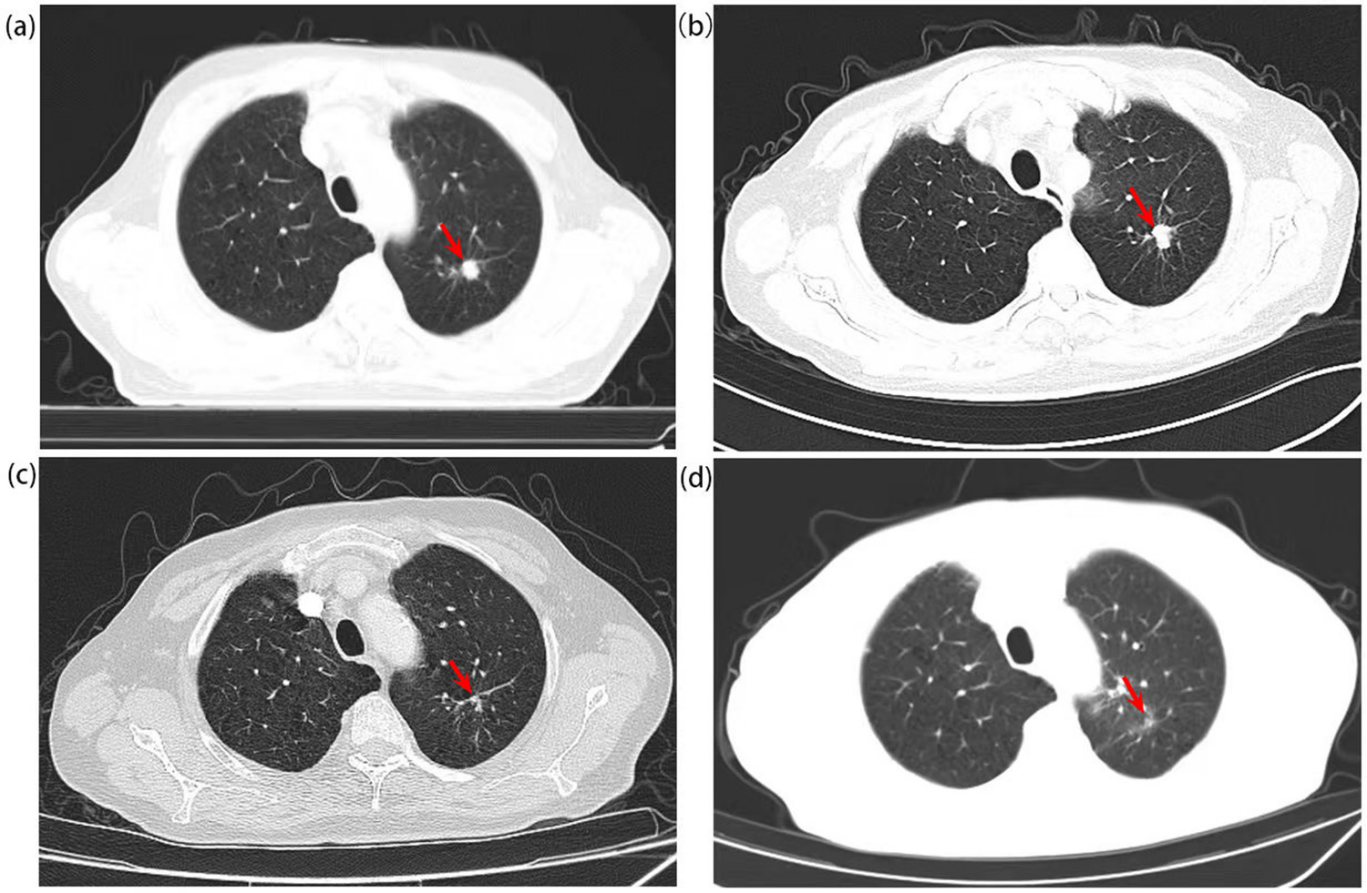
The CT scan of the chest shows the lung carcinoma: (a) shows a nodular hyperdense shadow seen in the posterior segment of the left upper lobe of the lung with medium contrast in August 2020 (with a size about 11 mm × 12 mm). (b) Shows the lesion before chemotherapy plus immunotherapy against PD-1. (c) Shows that the lesion was significantly reduced after two cycles of chemotherapy plus immunotherapy against PD-1. (d) Shows that the lesion almost disappeared after radiotherapy.
